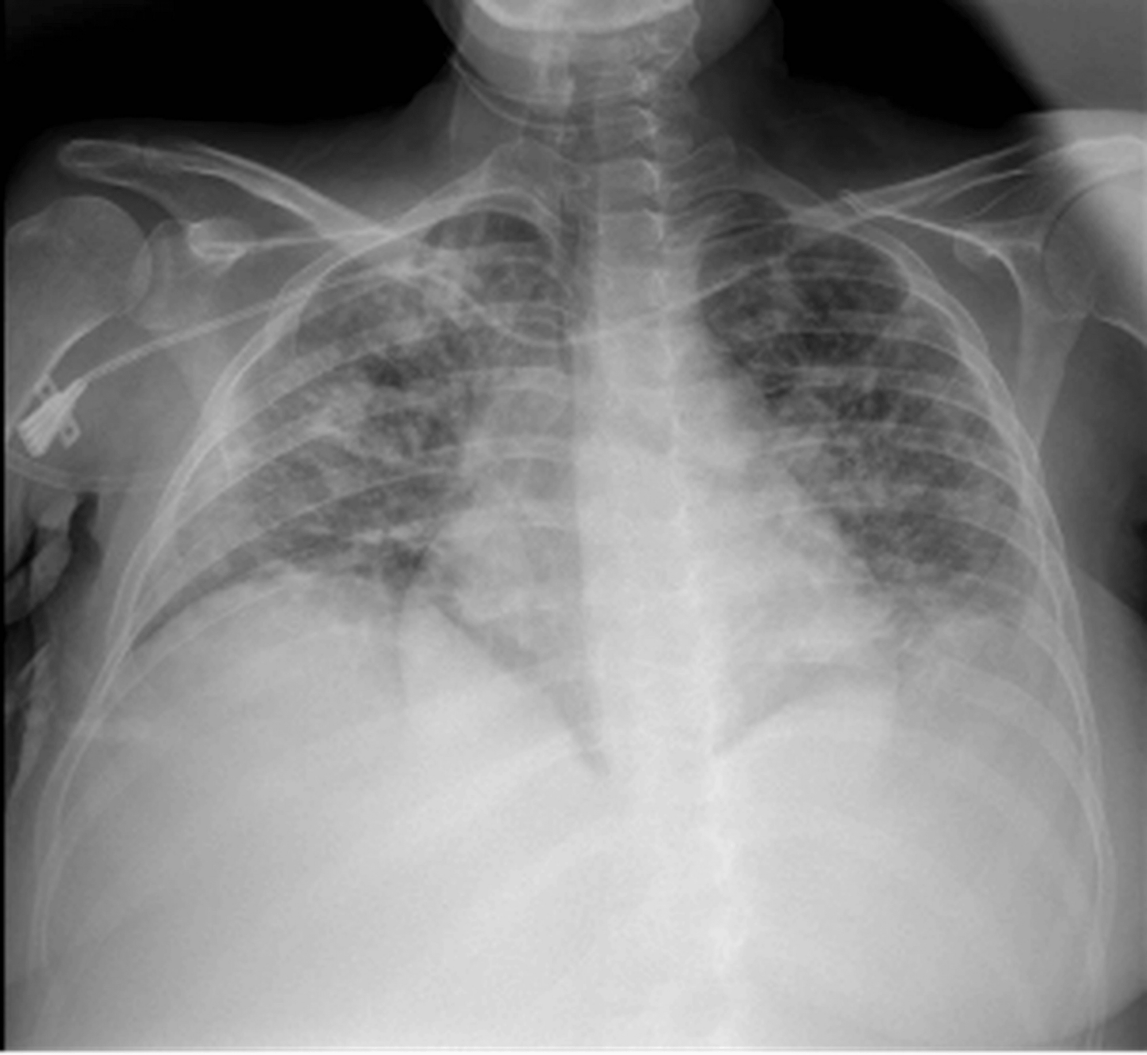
Imageological aspects of ARDS Thoracic X-Ray findings consistent with the diagnosis of ARDS, specifically bilateral diffuse alveolar opacities, with rapid progression. ARDS: Acute Respiratory Distress Syndrome
